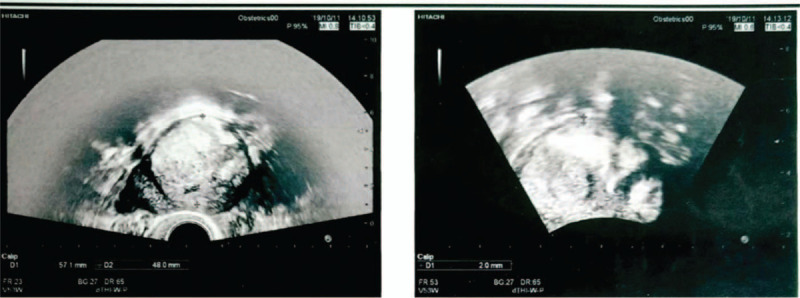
After more than 2 months of treatment, ultrasound indicated a 5.7 × 4.8 × 5.2 cm uneven echo group at the bottom of the uterine cavity, with a strong local echo and no obvious blood flow signal; the outer edge was about 0.2 cm from the thinnest part of the serous layer.
